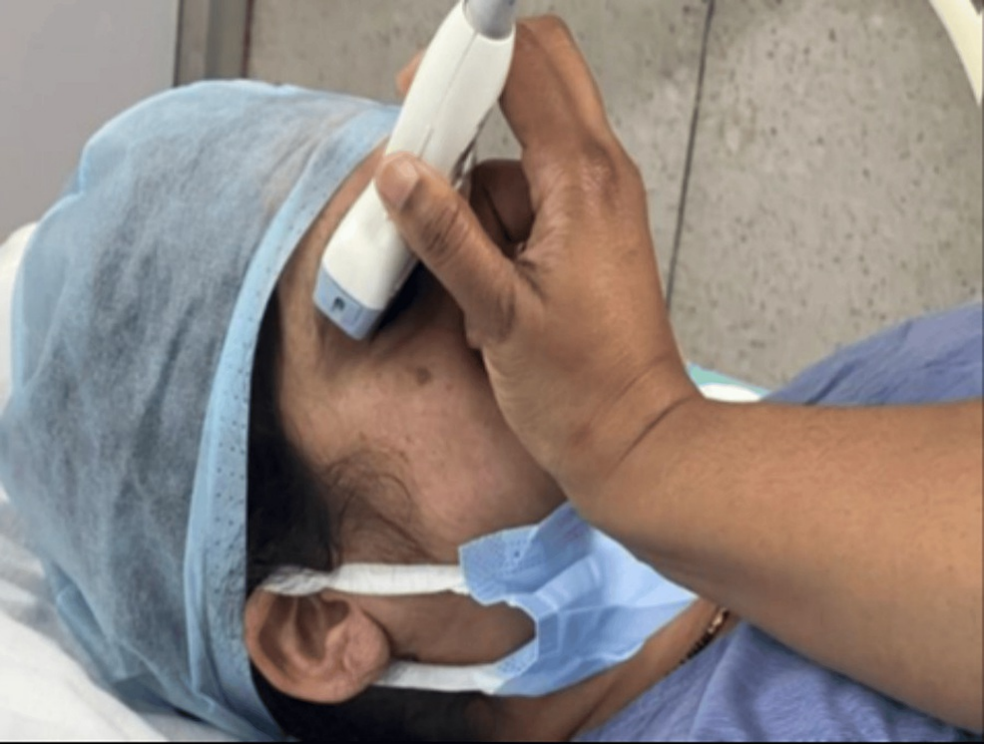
Placement of the ultrasound probe for the measurement of the optic nerve sheath diameter.
